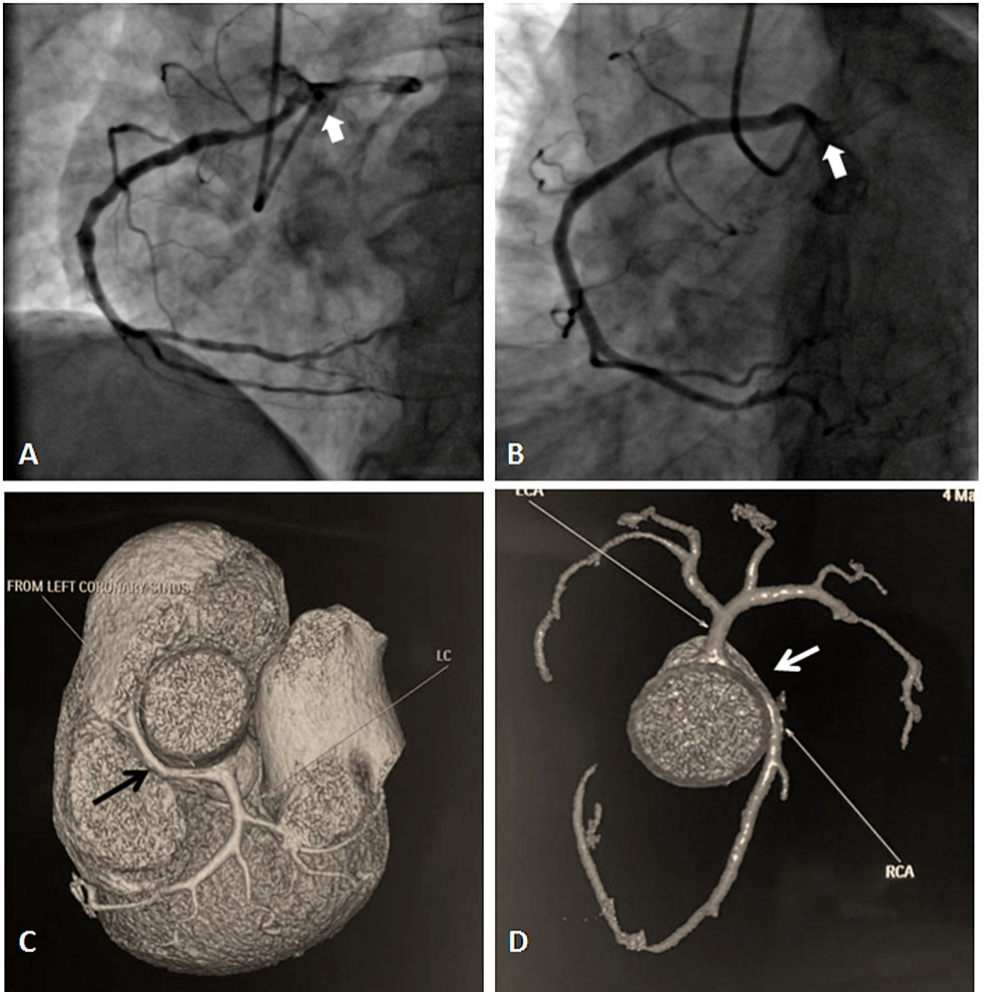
(A) and (B) shows the RCA arising from the left sinus; (C) and (D) CT images demonstrating inter-arterial course anomalous RCA (white arrow). LAO: left anterior oblique, RCA: right coronary artery.
